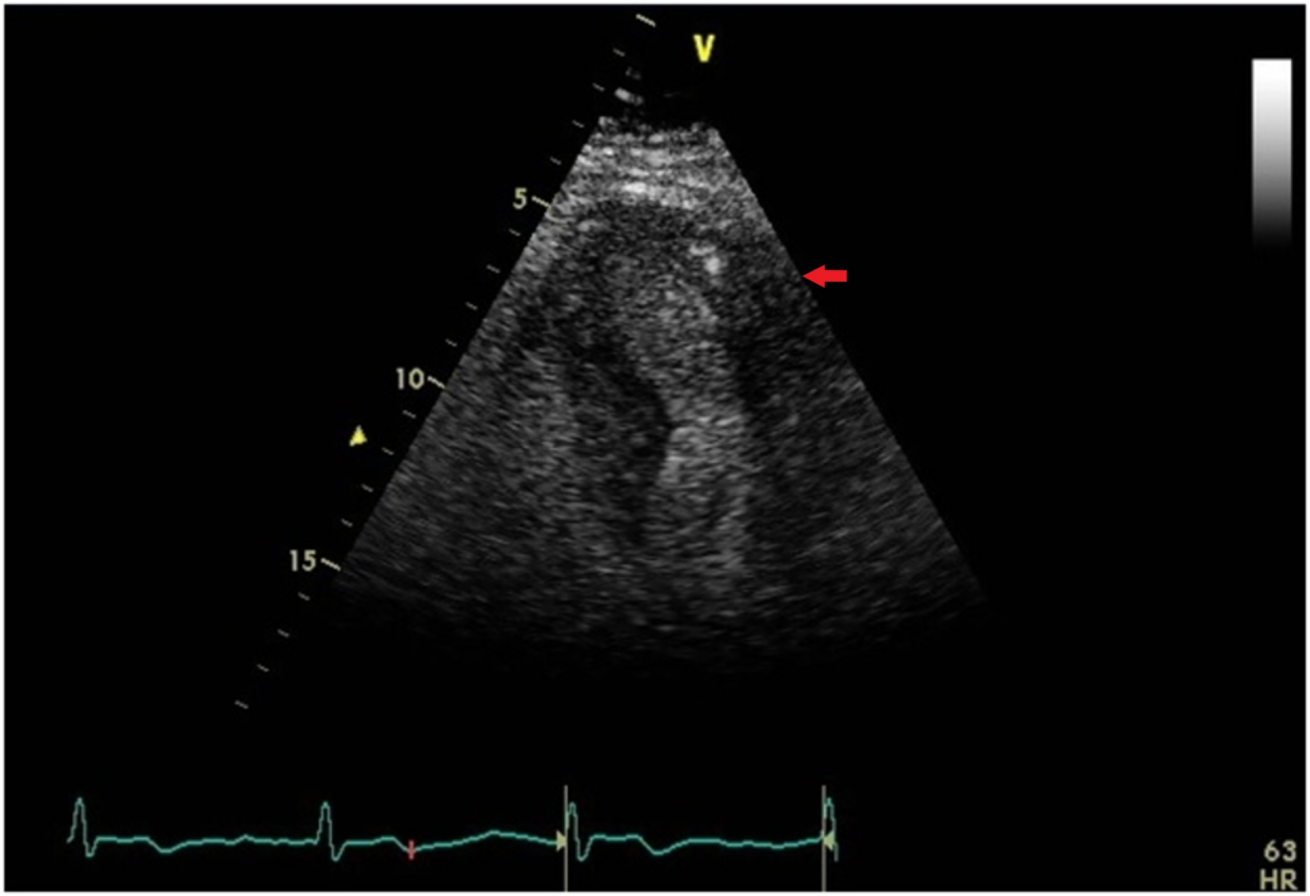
Apical four chamber view showing resolution of apical and periapical akinesia and dilation (red arrow).
